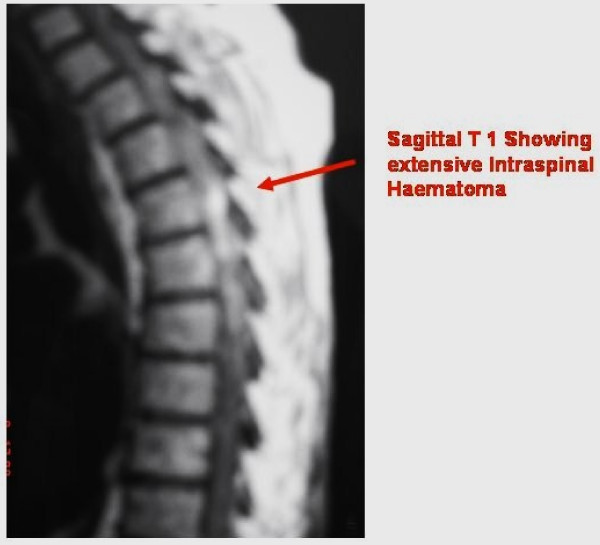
Pre-op MRI- T1 Sagittal showing extensive intra-spinal haematoma.
